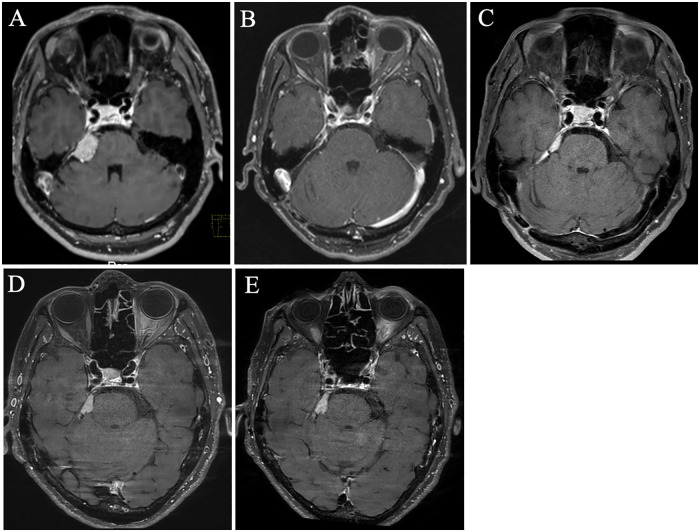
(A) Gadolinum-enhanced T1-weighted magnetic resonance imaging (MRI) at initial presentation shows a right petroclival meningioma. (B) Postoperative MRI demonstrates no residual tumor in the cerebellopontine angle. (C) Follow-up MRI at three years after surgery reveals slight tumor regrowth. (D) MRI 8 years after surgery, prior to Cyber Knife treatment, shows gradual tumor progression around the trigeminal nerve. (E) No significant tumor progression is evident on MRI at the time of the trigeminal neuralgia crisis, when compared to Figure D.
